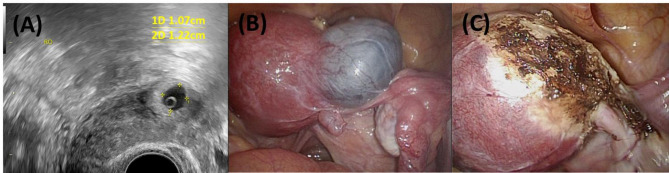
Imaging and surgical views of previous right interstitial pregnancy. A Transvaginal ultrasound image showing a right interstitial ectopic pregnancy. B Laparoscopic view of the right interstitial pregnancy before salpingectomy. C Laparoscopic view after salpingectomy, showing the surgical site post-resection
